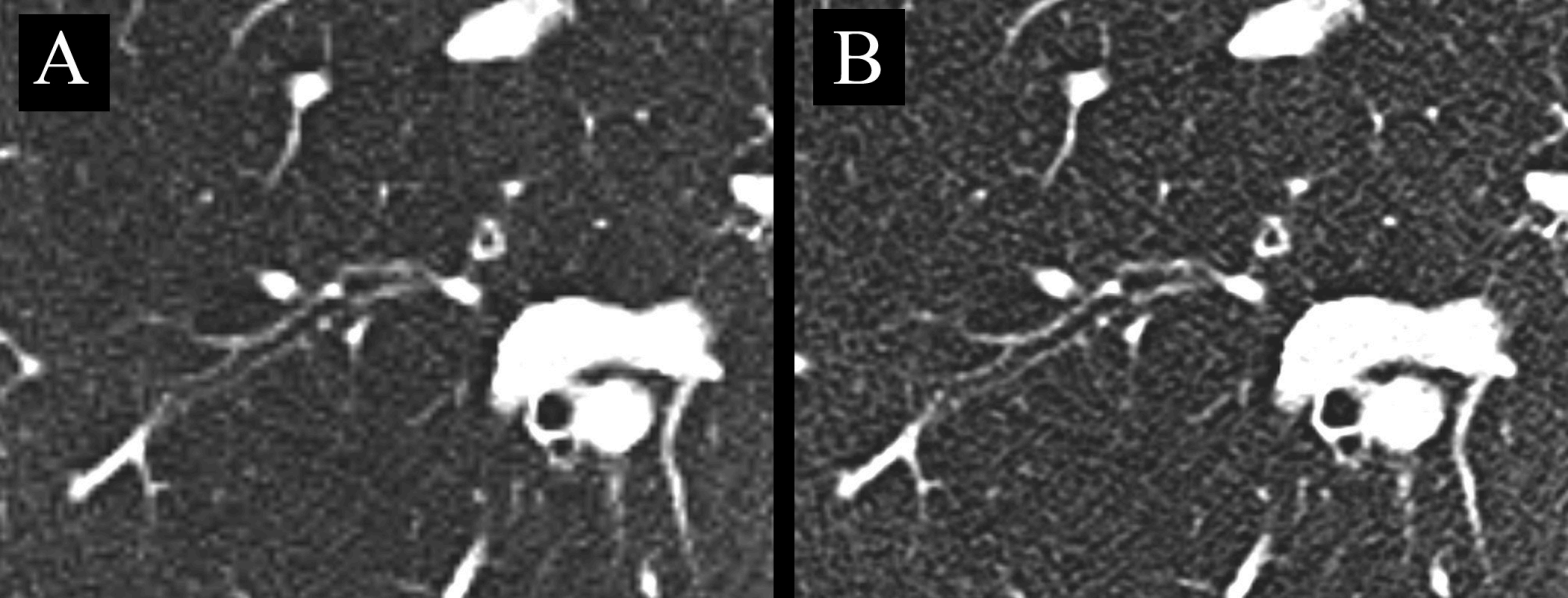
Image example of an axial CT slice of the same patient reconstructed with a 512-matrix (A) and 1024-matrix (B). Note the improved sharpness of the bronchial wall but increased image noise in B compared to A. Even though step artifacts are present in A, readers gave similar ratings for overall image quality of bronchial structures.
